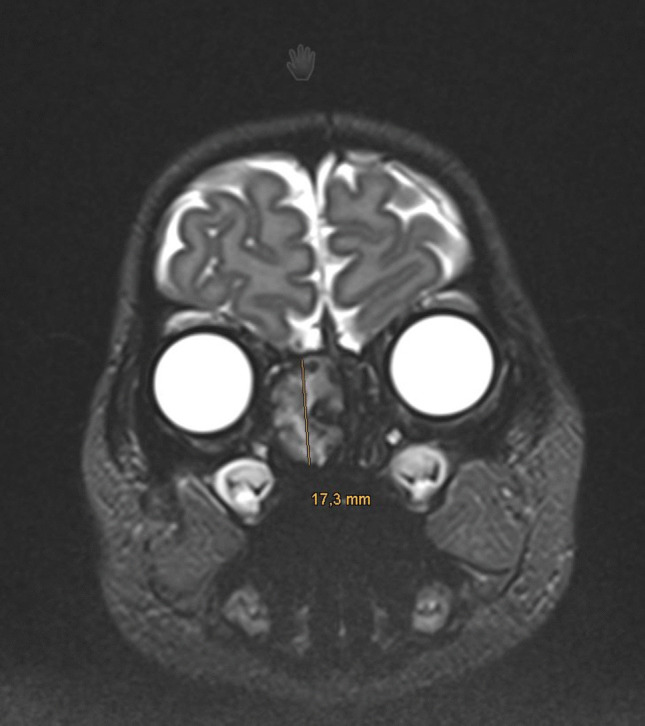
Expansion of Kaposiform Haemangioendothelioma in T2 weighted MRI scan in coronal plane
